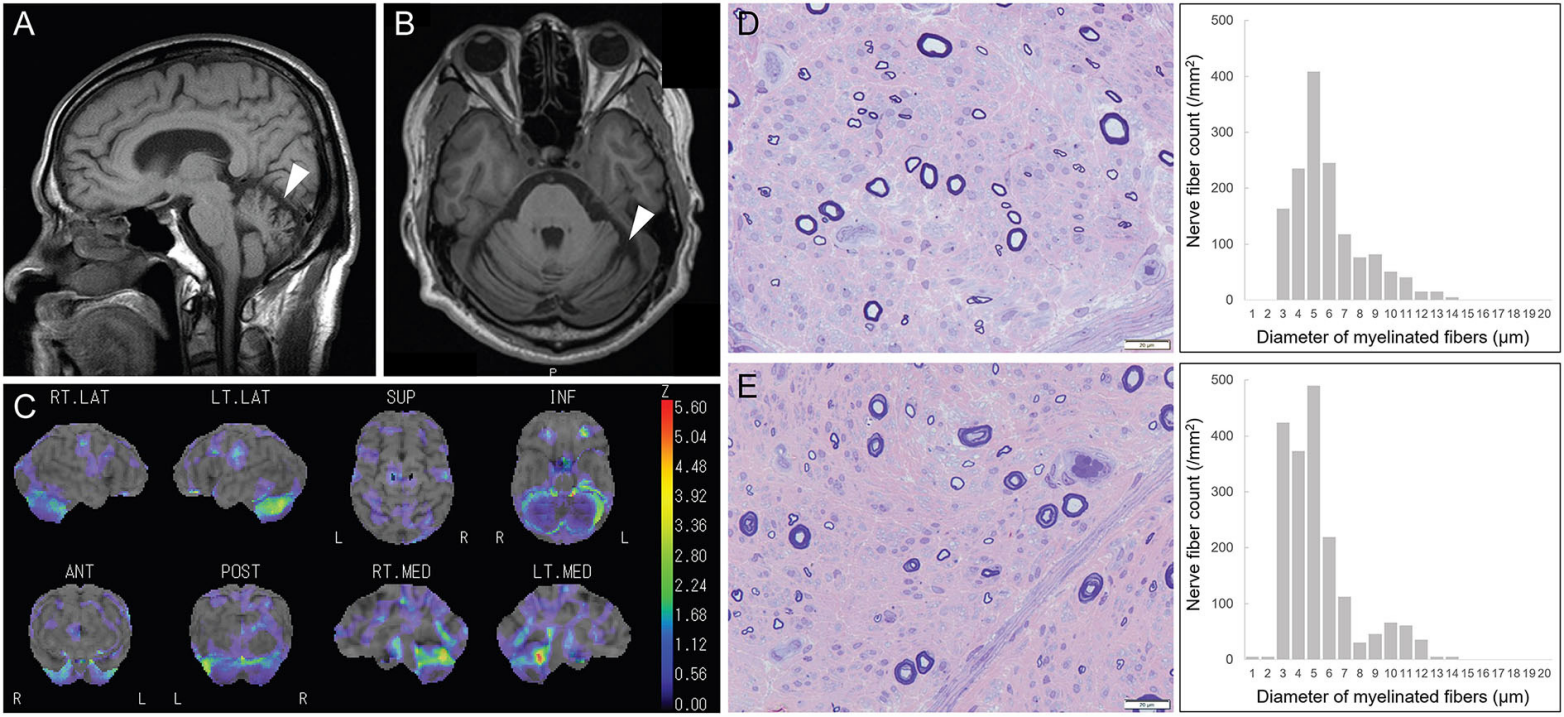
Radiological and pathological features of patients with disease-associated *RFC1* repeat expansions. (A,B) Brain MRI shows cerebellar atrophy (white arrow; P10). (C) Brain single photon emission computed tomography (SPECT) reveals decreased blood flow in the cerebellar region (P21). (D,E) Marked loss of large and small myelinated fibers (large > small). Myelinated fiber densities are 1,456/mm^2^ and 1,880/mm^2^, respectively, from P31 with [(AAGGG)exp/(AAGGG)exp] and P10 with [(ACAGG)exp/(ACAGG)exp].
